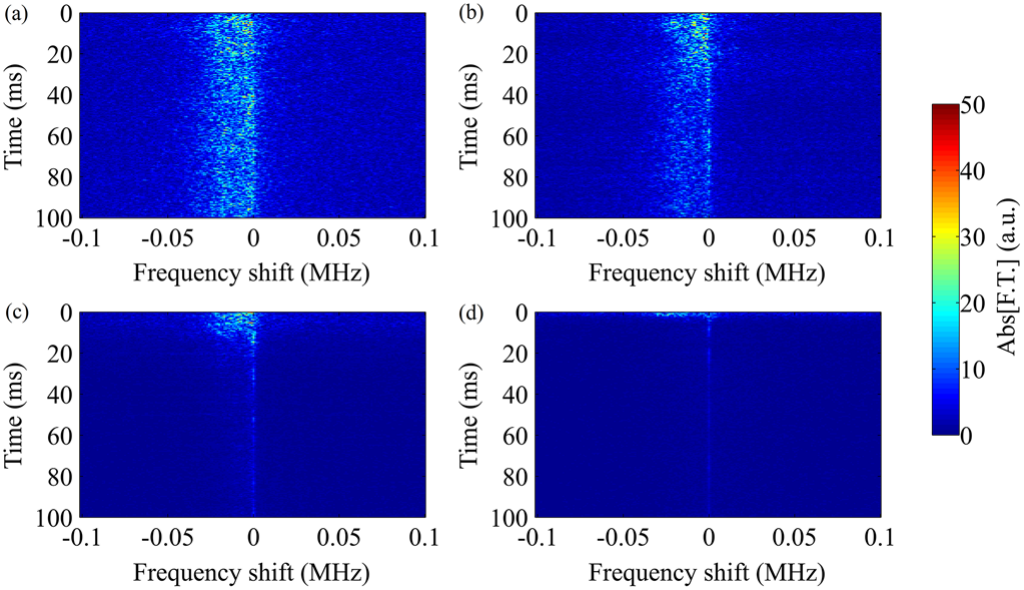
Time-resolved Doppler shift for different microbubble concentrations. (a) 5  ×  10^7^, (b) 5  ×  10^6^, (c) 5  ×  10^5^, and (d) 5  ×  10^4^ microbubbles ml^−1^ (10th harmonic, PRP: 217 kPa).
